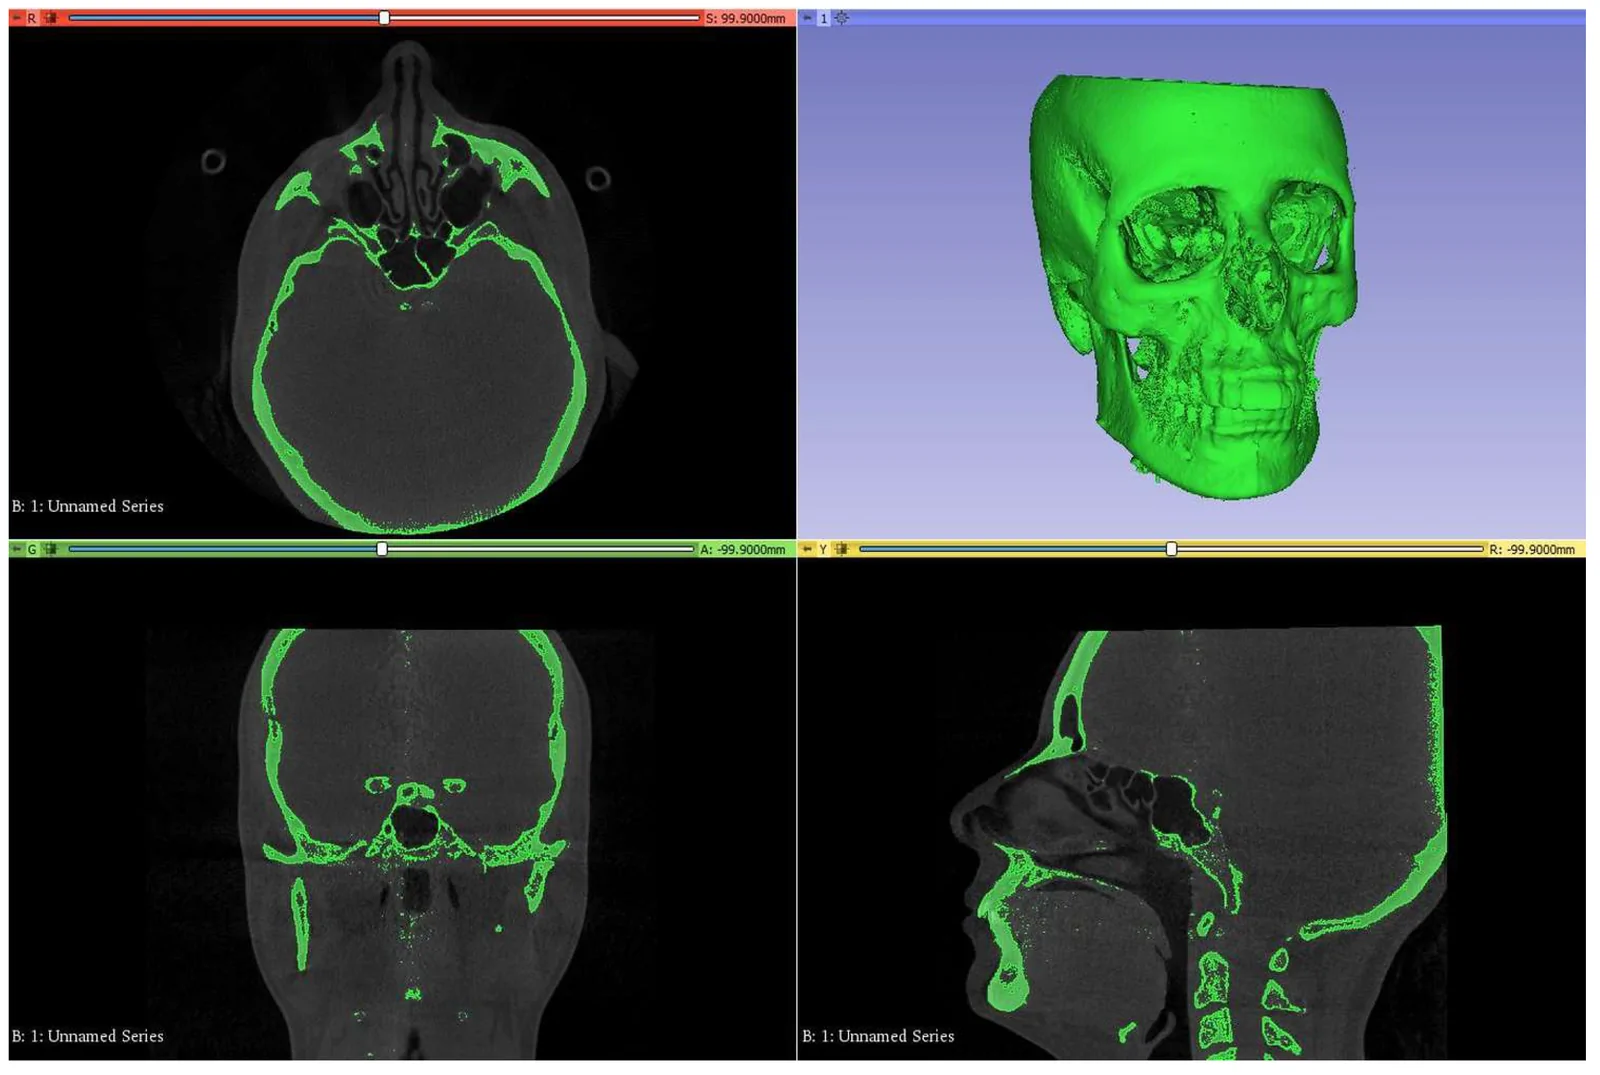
Generation of 3D anatomical models from the Visible Human Project CT dataset.
